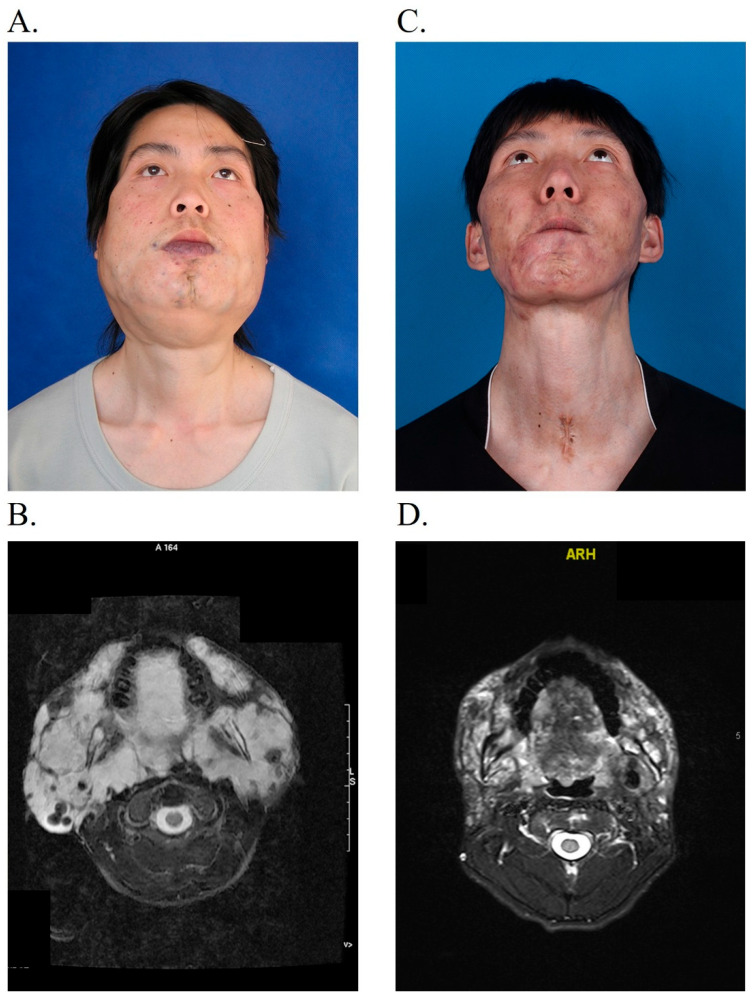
A 22-year-old man with a huge venous malformation in his face and neck. (A) The patient had his face, neck, and respiratory tract surrounded by tissue affected by VM. (B) MRI images show the lesion located in the surrounding tissue of the respiratory tract. The parapharyngeal space, tongue, and soft palate are involved. (C,D) Thirty-eight months later, after the tracheotomy tube was removed and the sclerotherapy was finished. Both the volume and extent of VM were evidently diminished.
